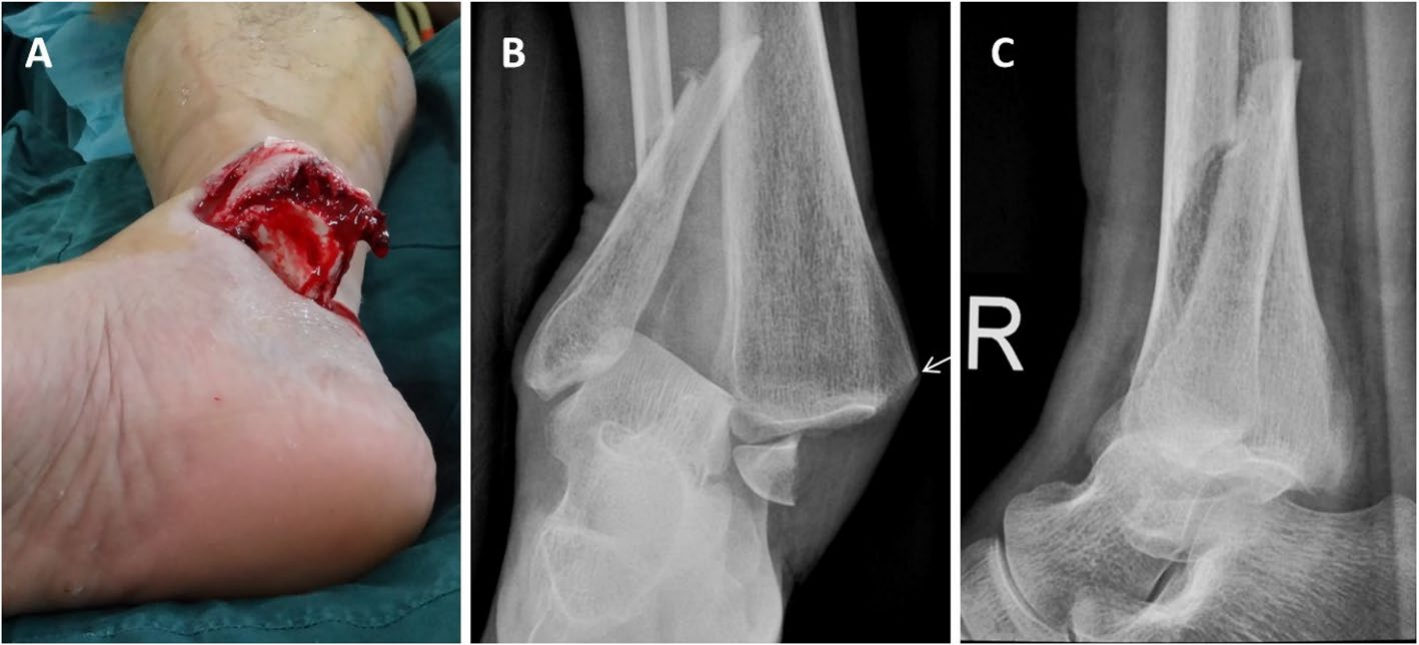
A Medial open wound of the ankle joint and bone exposure of the distal tibia. B,C Anteroposterior and lateral X-ray films of the ankle with pronation-external rotation closed Logsplitter injury, with the visible continuous and complete medial soft tissue of the ankle joint displayed on the anteroposterior X-ray film (arrow)
